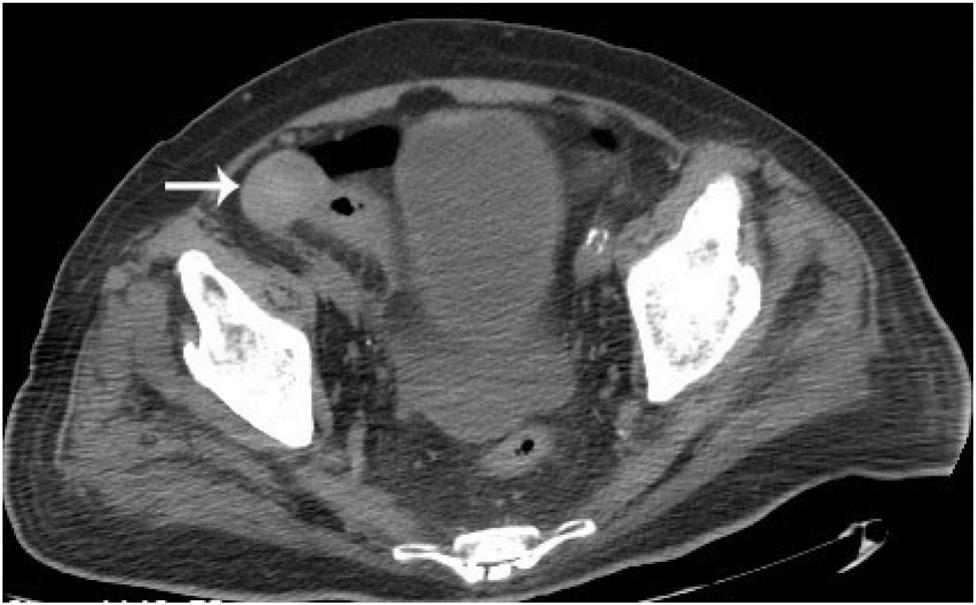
The peritoneal loose body in the abdominal CT scan after bowel perforation occurred.
